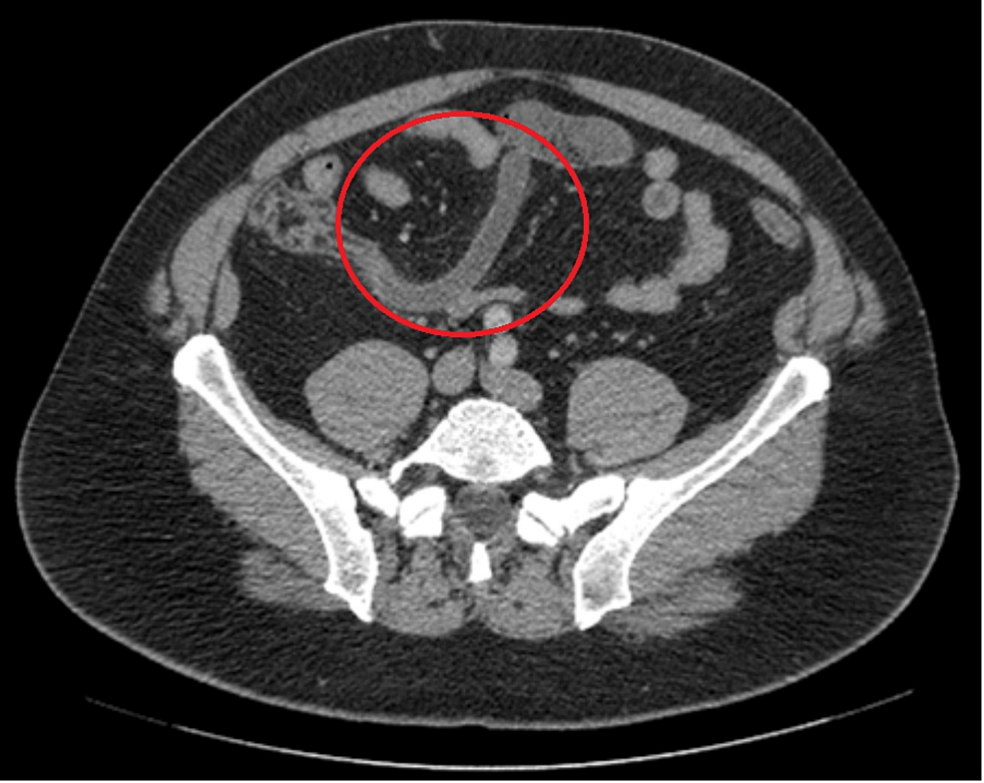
Appendicitis captured on computed tomography transverse view prior to appendectomy procedure
